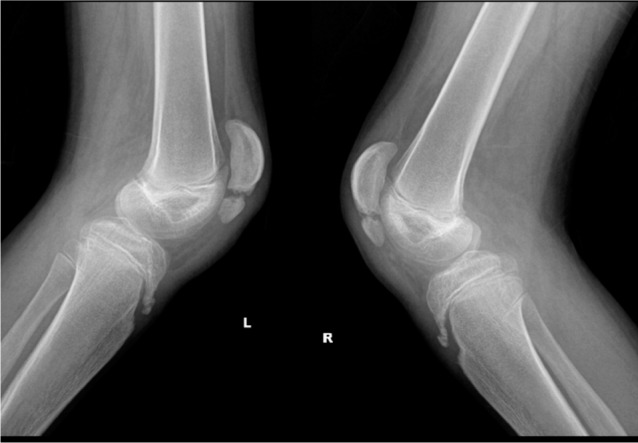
Pre-op radiograph of knee showing fixed flexion deformity with patella alta with fragmentation of distal part of patella.
